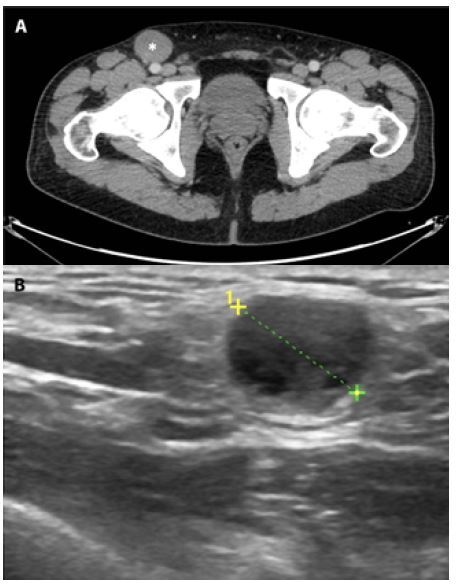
Lymph node metastasis of cutaneous squamous cell carcinoma from a primary tumor on the right sole. (A) CT-scan shows a well defined 20 mm nodule on the right superficial groin (white asterisk). (B) Regional ultrasound showed an anecoic rounded structure. The patient underwent a groin lymph node dissection.
